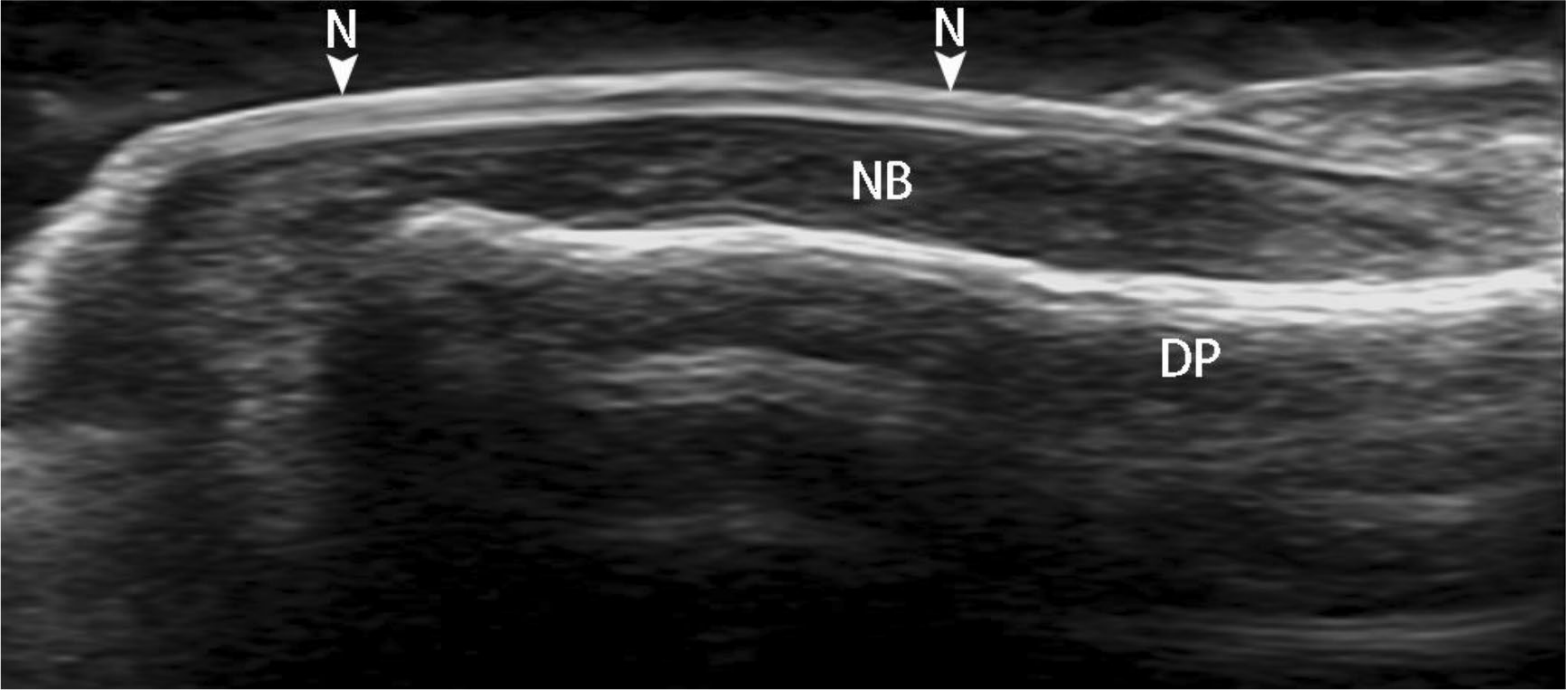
High resolution (frequency) ultrasound shows the normal dorsal anatomy of thumb, DP = cortex of distal phalanx, N = nail, NB = nail bed.
